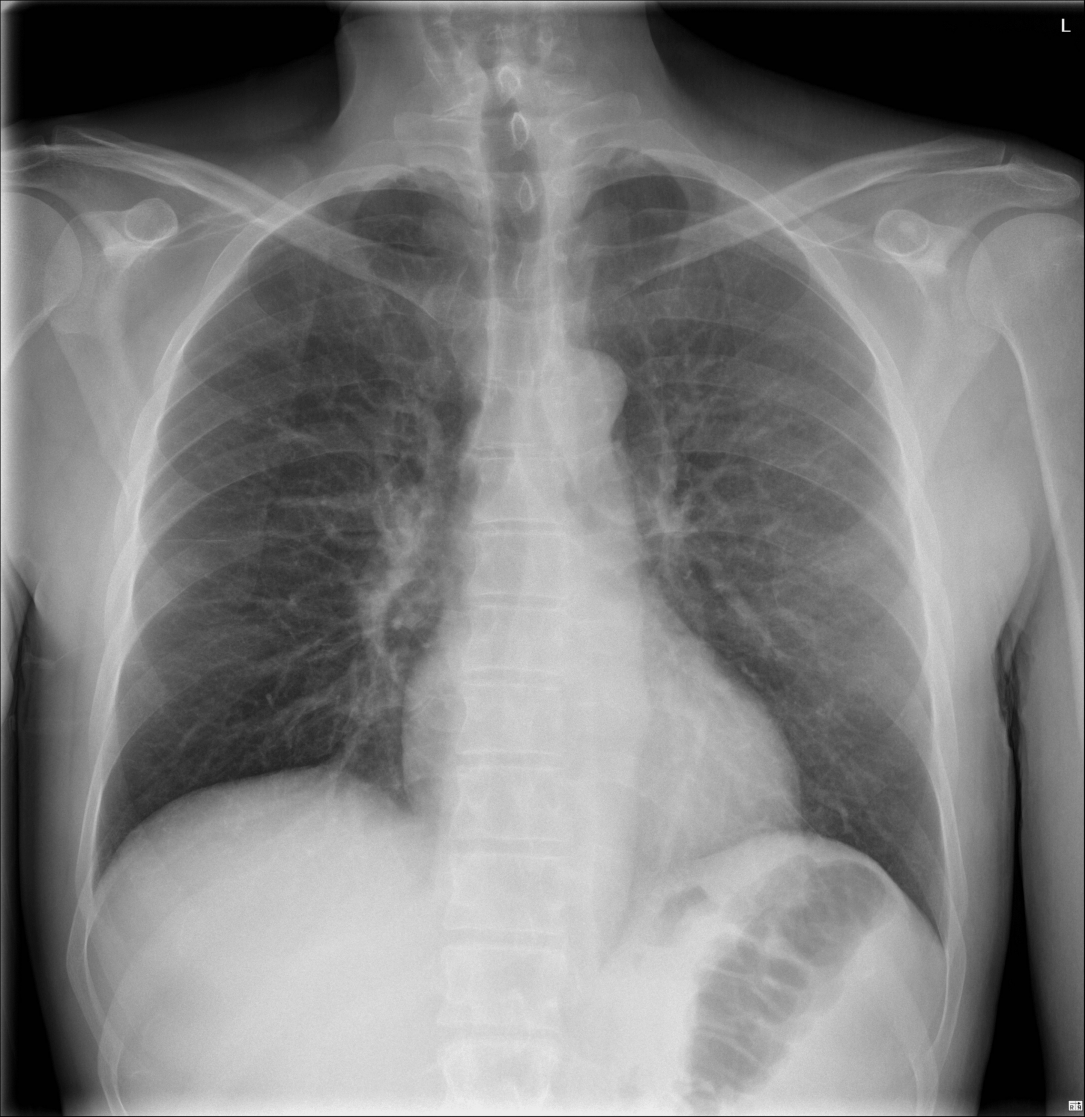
Chest posteroanterior X-ray showing no evident lesions suggestive of right-sided valvular involvement.
